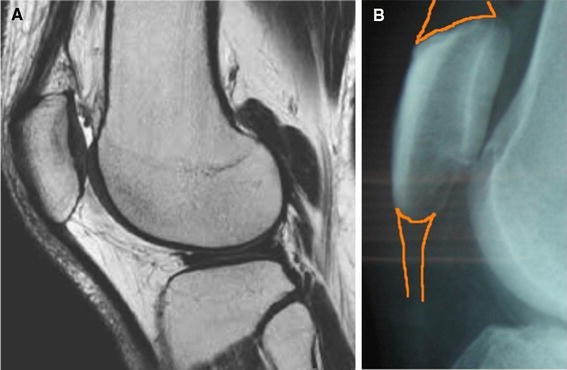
Relationship between the patella and quadriceps femoris and patellar tendons. a MRI, b fluoroscopy: the quadriceps femoris tendon is attached anteroposteriorly to the patella widely at the superior margin but narrow at the inferior margin
